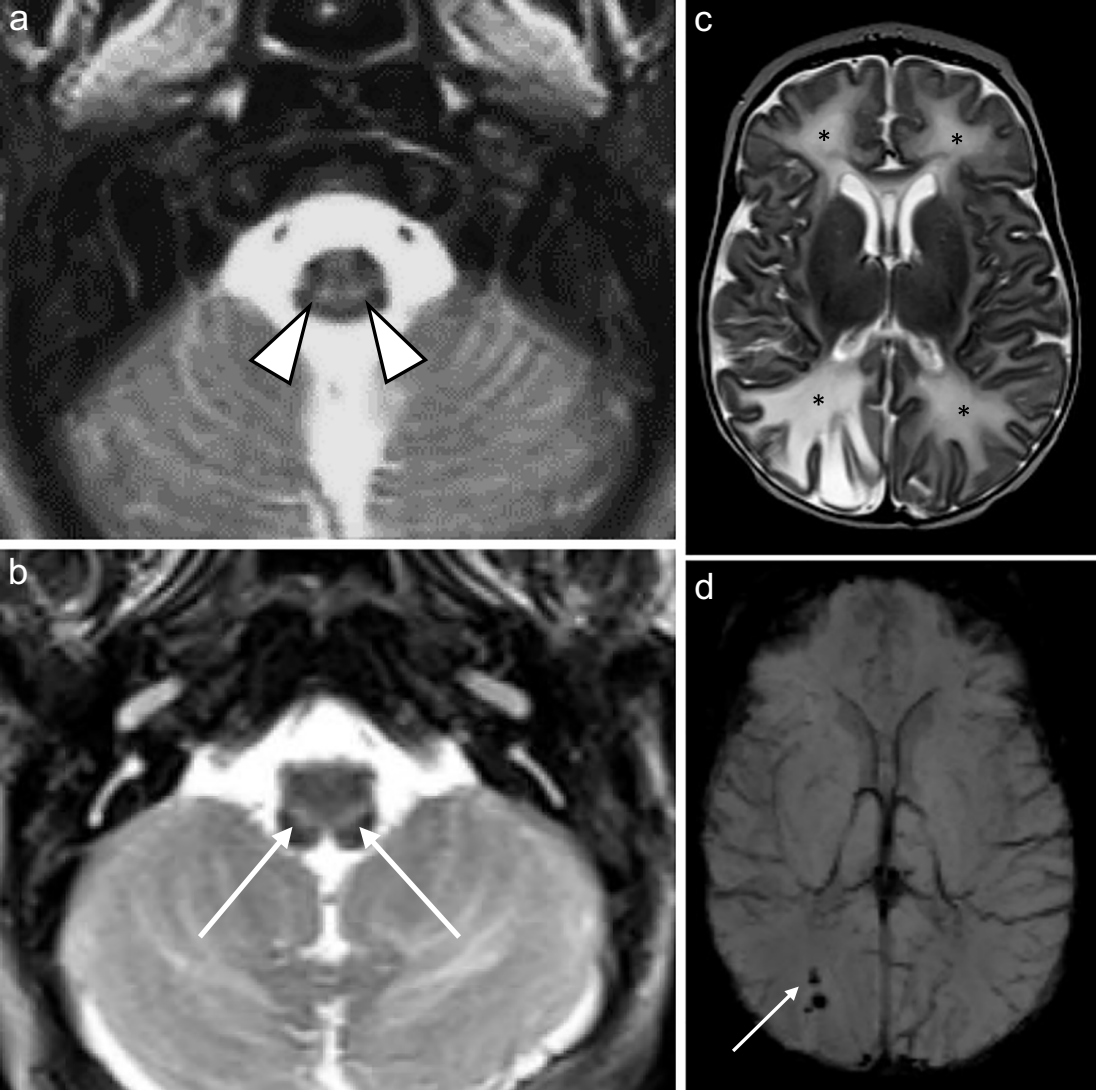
A 5-week-old girl with genetically confirmed *MPV17*-related mitochondrial depletion syndrome. a Axial T2-weighted image shows hyperintense signal in the reticulospinal tracts of the proximal cervical cord (*arrowheads*). b Axial T2-weighted image shows hyperintense signal in the reticular formation of the medulla (*arrows*). c Axial T2-weighted image shows extensive white matter disease involving the periventricular, deep, and subcortical white matter with extension into the U-fibers (*asterisks*). d Susceptibility-weighted imaging reveals blooming at the site of maximally diseased white matter (*arrow*)
